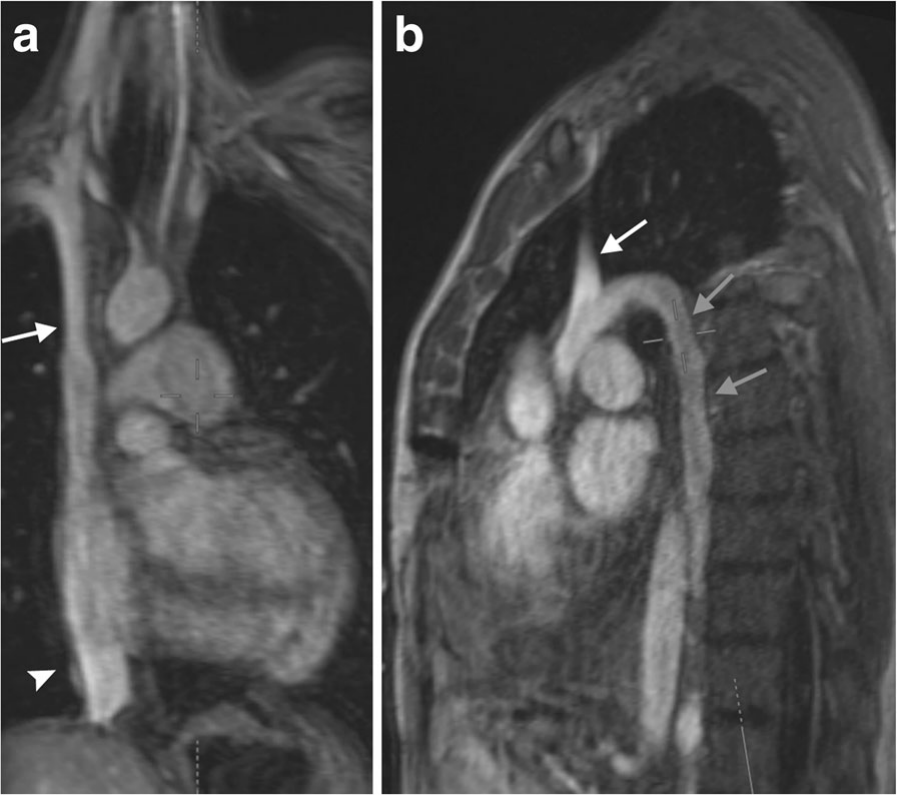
MRI venous imaging. Coronal (a) and sagittal (b) images of the thoracic venous anatomy in the late arteriovenous phase of imaging of a MRI scan. The SVC (white arrow), IVC (arrowhead), and azygos vein (gray arrows) are labeled
